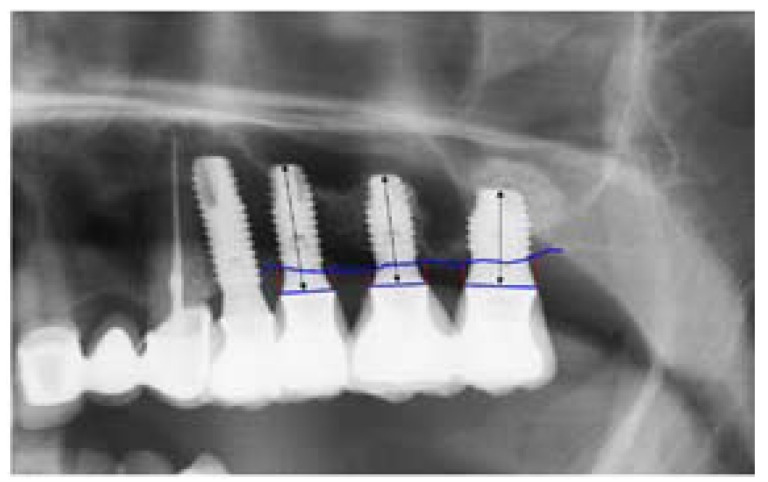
Postoperative panoramic X-ray view. Measurement of bone loss after prosthetic loading.
